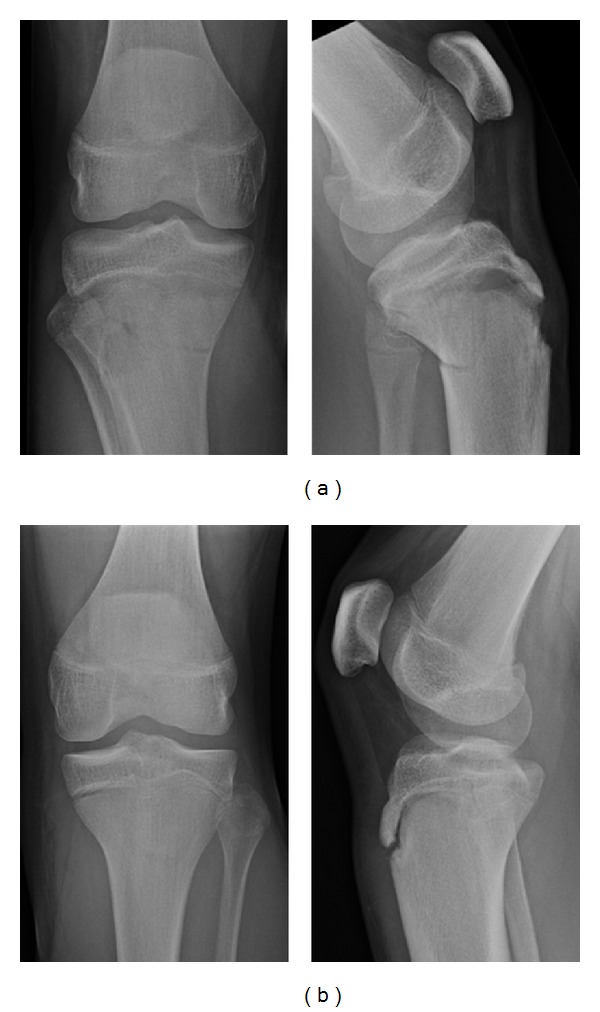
Plain radiographies of the right (a) and left knee (b) after trauma revealed transitional fractures of the proximal tibia, on the right side dorsally displaced, on the left side undisplaced.
